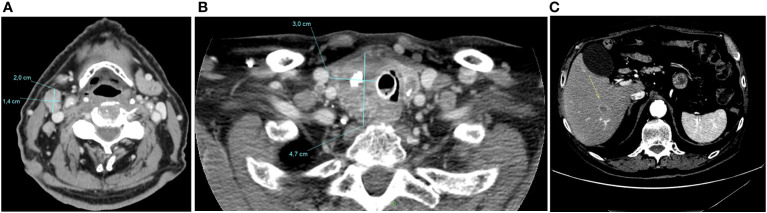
CT-scan performed in March 2021, after four months of Lenvatinib, showing a dimensional reduction of latero-cervical lymphadenopathies (maximum diameter of 2 cm) (A), and of left thyroid lobe (3x4,7 cm) (B), and a mild increase of the hepatic lesion (17 mm vs 12 mm) (C).
